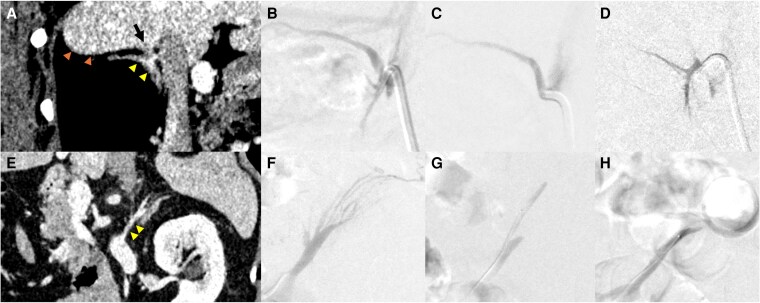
Adrenal imaging and venous angiography of representative cases. A to D and E to H show representative images of computed tomography (CT) and venous angiography from a 56-year-old and a 55-year-old man diagnosed with primary aldosteronism (cases 1 and 2), respectively. In case 1, preoperative CT revealed a confluence of the right adrenal vein and hepatic vein (A, yellow and orange arrowheads indicate the right adrenal and the liver, respectively. A black arrow marks the accessory hepatic vein, which flows into the right adrenal vein). B, Adrenal venous sampling (AVS) confirmed this confluence. The right adrenal and hepatic veins were separately selected by a catheter (C and D, respectively) and the venous partial pressure of oxygen levels at baseline were measured as 69.9 and 45.1 mm Hg, respectively. In case 2, enhanced CT visualized the left adrenal vein (E, yellow arrowheads). F, AVS identified the left adrenal vein with 3 main branches. After exclusion of the proximal one as the inferior phrenic vein, the middle and distal branches were individually cannulated for blood sampling (G and H, respectively), confirming the distal one as the left adrenal central vein based on the selectivity index. The blood partial pressure of oxygen level at baseline was measured as 37.1 mm Hg in the middle vein and 57.7 mm Hg in the distal vein.
